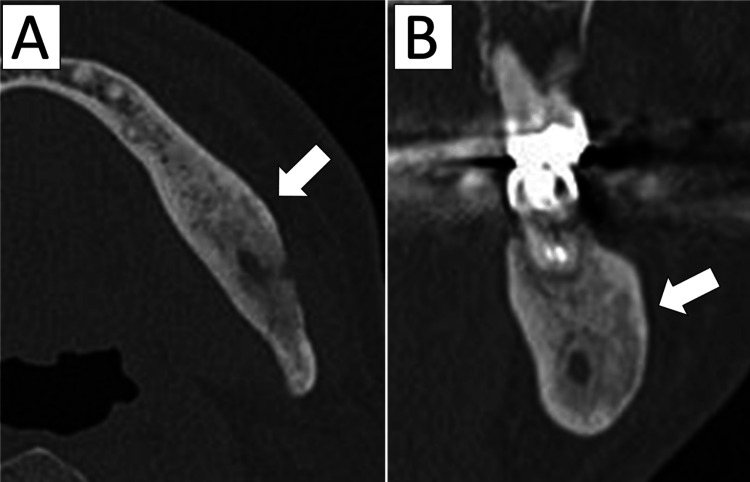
CT six months after BP therapy A, B: Although some radiolucent areas remain, ossification is observed in most of the previously osteolytic regions (arrows) BP: Bisphosphonate
